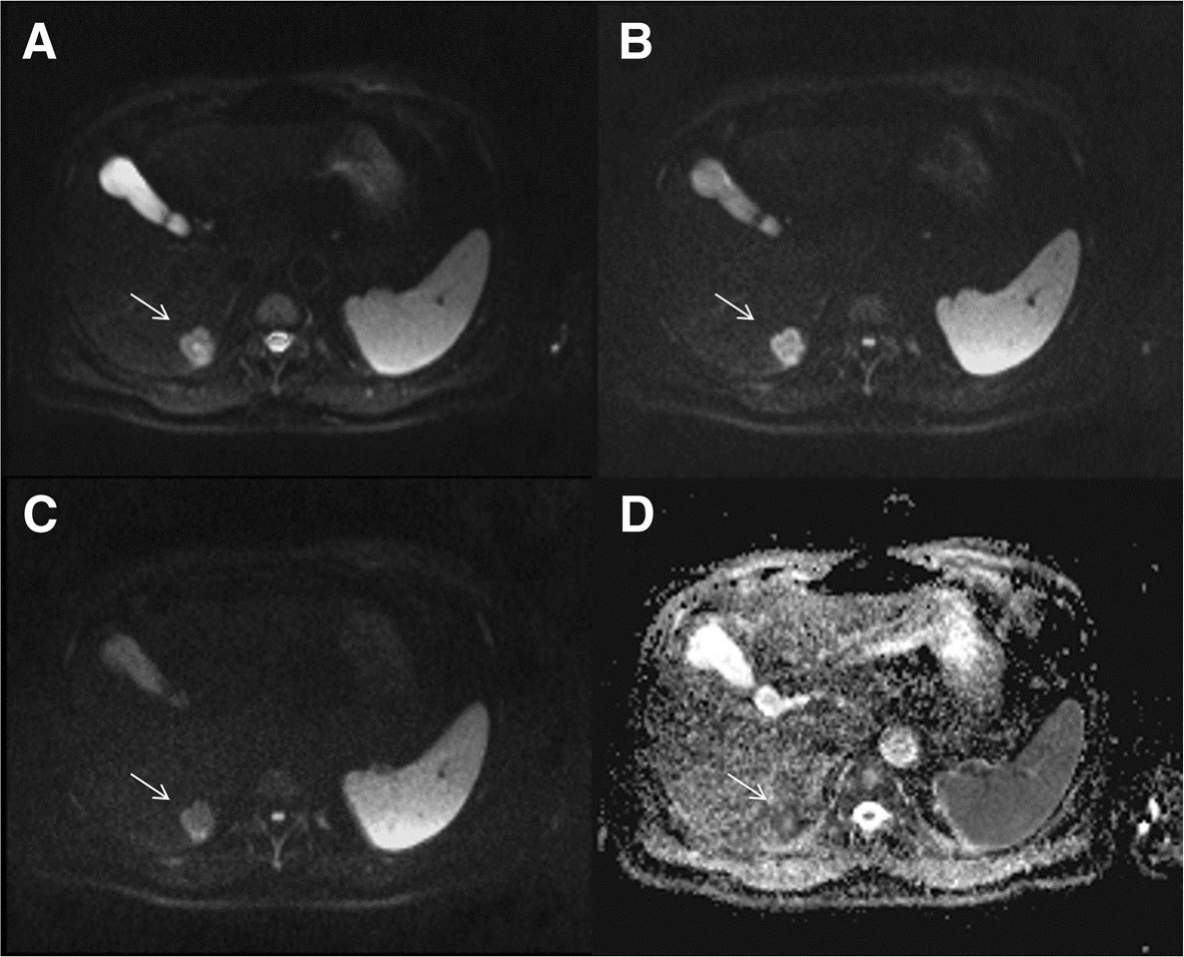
The same patient of Fig. 1. DWI sequences (a: b50 s/mm^2^; b: b600 s/mm^2^; c: b800 s/mm^2^; d: ADC map). The lesion (arrow) shows a restricted diffusion for all b values. The ADC value is 1.18 × 10–3 mm^2^/s
